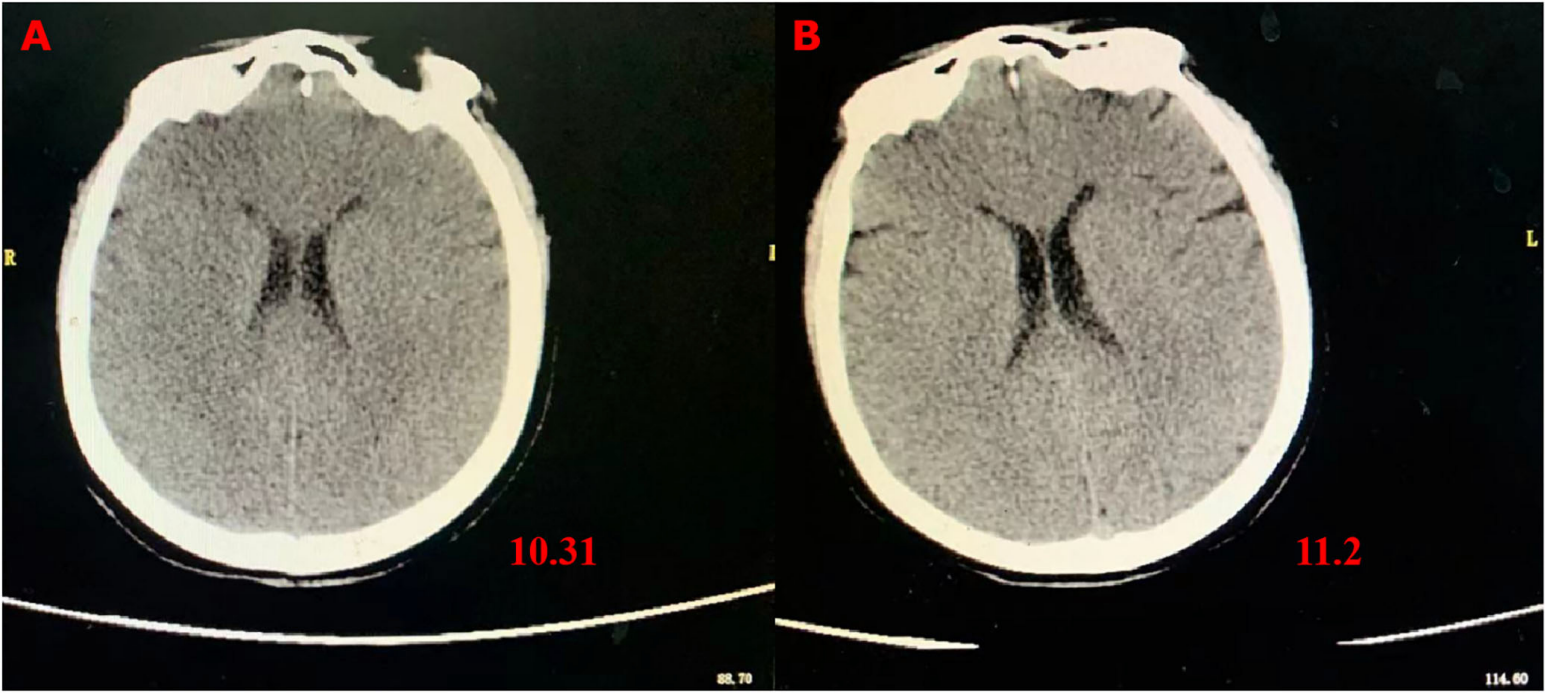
Skull CT images showing brain edema. (A) CT scans at 72 h after admission; (B) CT scans on the fourth day after transferring out of the ICU.
